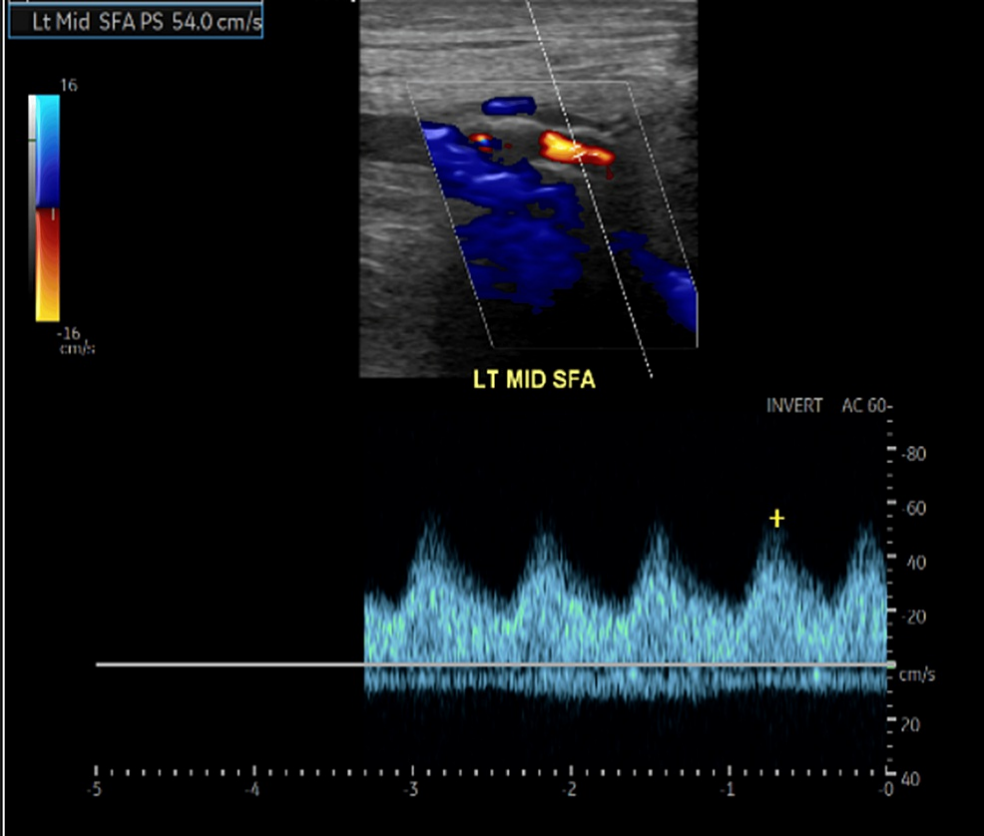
Duplex arterial ultrasound of monophasic waveforms in left mid-SFA (superficial femoral artery)
